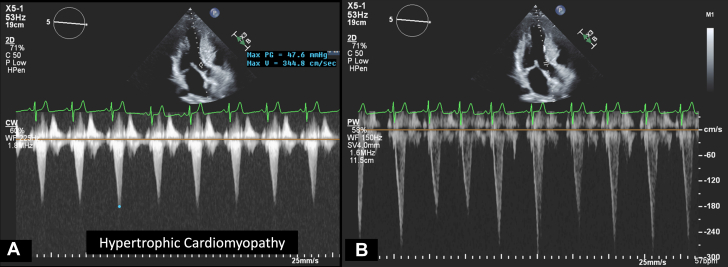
Doppler Profiles of a Patient With Hypertrophic Cardiomyopathy Continuous-wave Doppler of a patient with hypertrophic cardiomyopathy showing late peaking dagger-shaped CW Doppler waveform (A) and PW Doppler waveform showing increased velocity with aliasing (B).3.Poor alignment of your Doppler cursor will lead to underestimation or overestimation of gradients. Improper placement of PW sample volume will overestimate (if too close to AV) or underestimate (if too far from AV) the left ventricular stroke volume, leading to inaccurate AV area measurement using the continuity equation. When positioning the PW Doppler sample volume in the LVOT, if spectral broadening artifact is occurring, reposition the sample volume away from the flow convergence. Alignment of the CW Doppler cursor as parallel to the direction of blood flow will maximize the AV velocity and gradients. In patients with heavily calcified AV, bicuspid AV, or tortious aortas, alignment of the CW cursor may be challenging. Using color Doppler can help identify the direction of blood flow and then best align the CW Doppler cursor. It is important to attempt imaging in all recommended views using both imaging and Pedoff (nonimaging) probes.4.Ultrasound enhancing agents can be used to obtain gradients across the AV but require careful gain adjustments to avoid overestimation of the Doppler profile (Figure 18).
